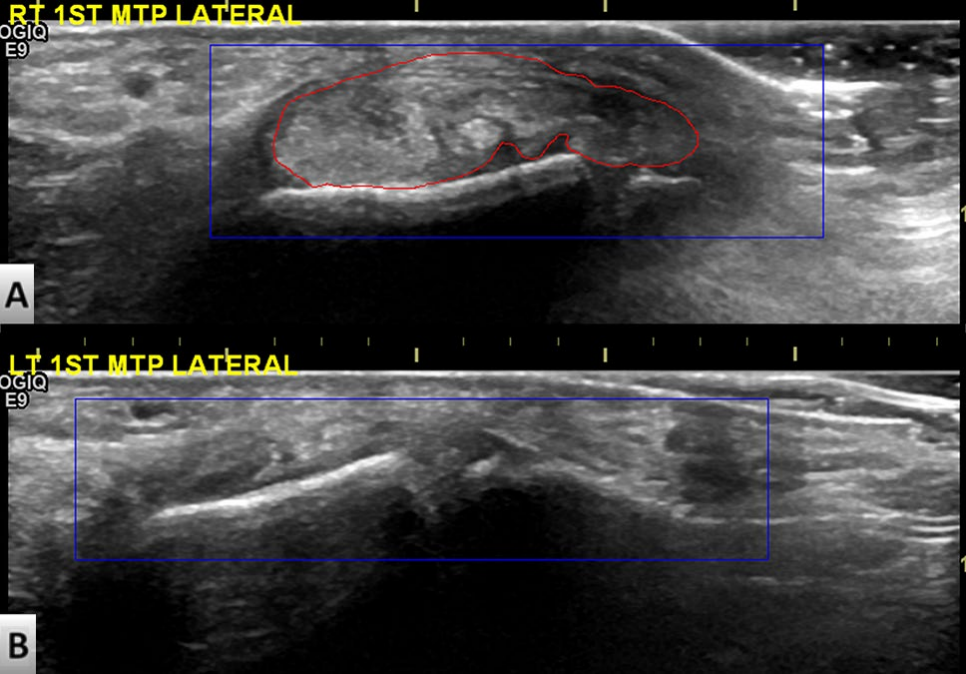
Ultrasound images of (A) left 1st metatarsophalangeal joint lateral side with tophi and (B) right 1st metatarsophalangeal joint lateral side without tophi in single gout patient. The red curve indicates the boundary of the tophi region. The blue rectangle frame indicates the region of interest drawn by a rheumatologist for subsequent transfer learning.
